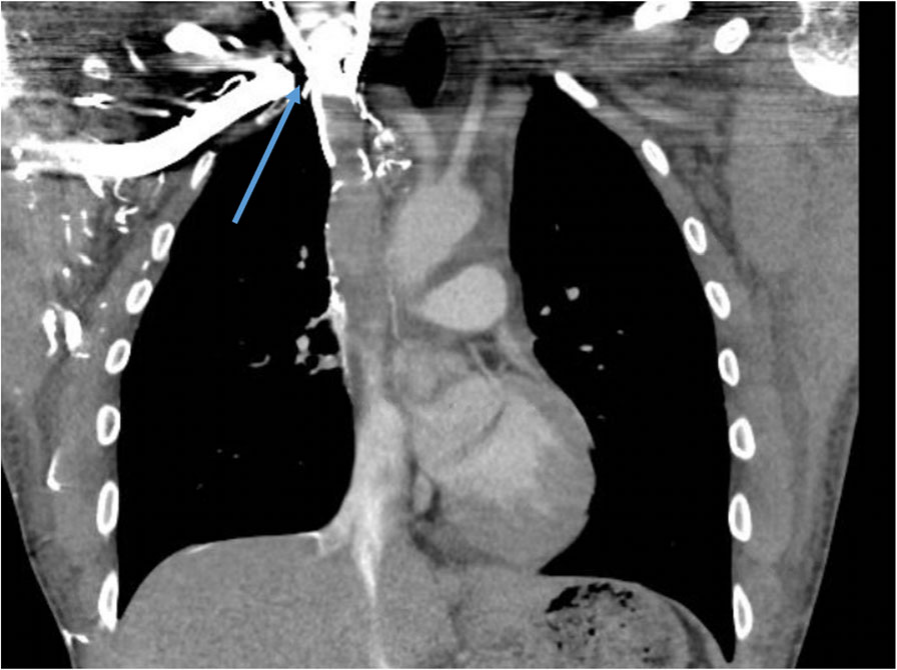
CT chest on admission showed SVC thrombus that extended to involve the brachiocephalic vein
